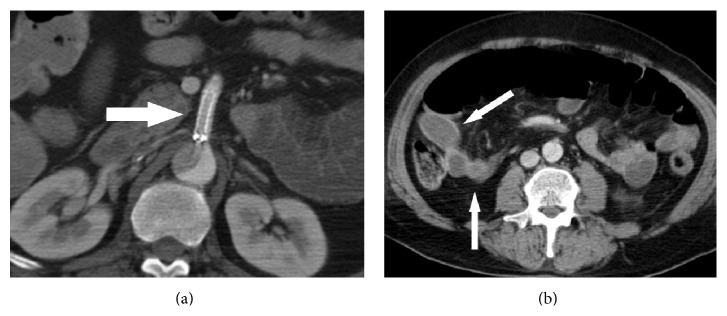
Contrast-enhanced CT shows (a) the patency of the stent placed in the SMA (arrow) and (b) the improvement of enhancement of the small intestine (arrow).
